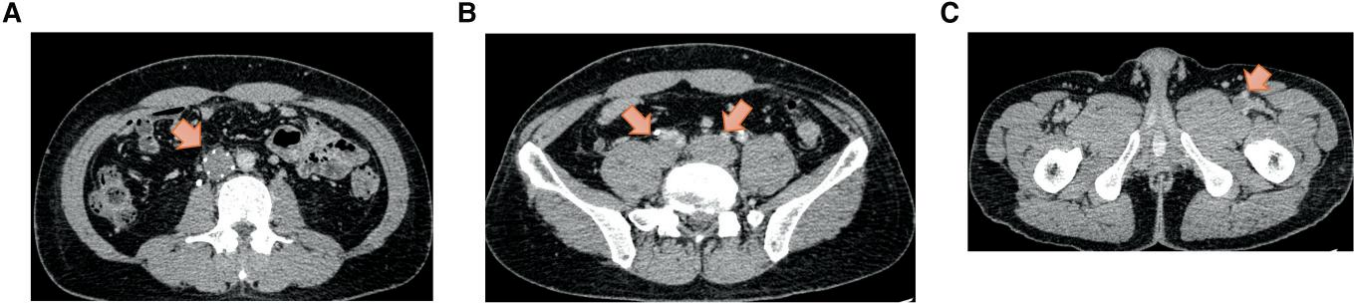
Findings on computed tomography images obtained on hospital Day 37. (*A*–C) Images showing a thrombus extending from the left femoral vein into the inferior vena cava filter (arrows) despite anticoagulation therapy with heparin.
